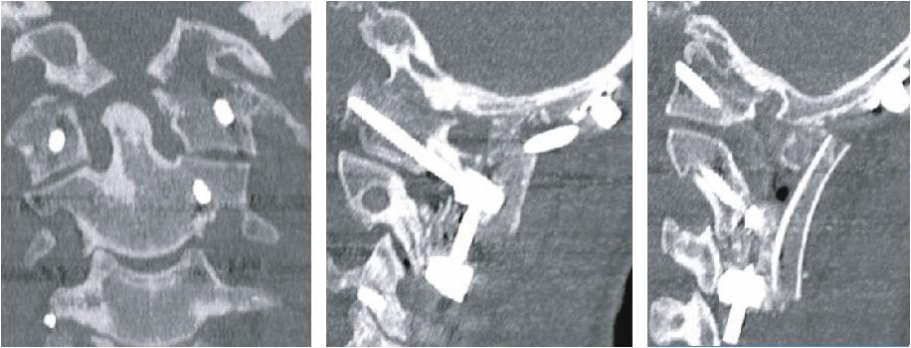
Case 2: CT postoperative. Anatomic alignment with reduction of the atlantooccipital fracture dislocation with bilateral C1, C2, and C3 lateral mass screws.
